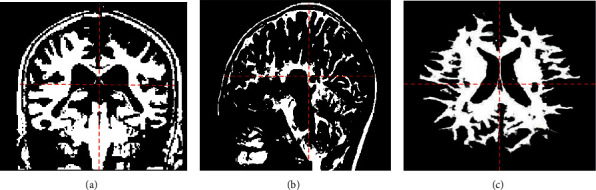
MRI image registration example.
